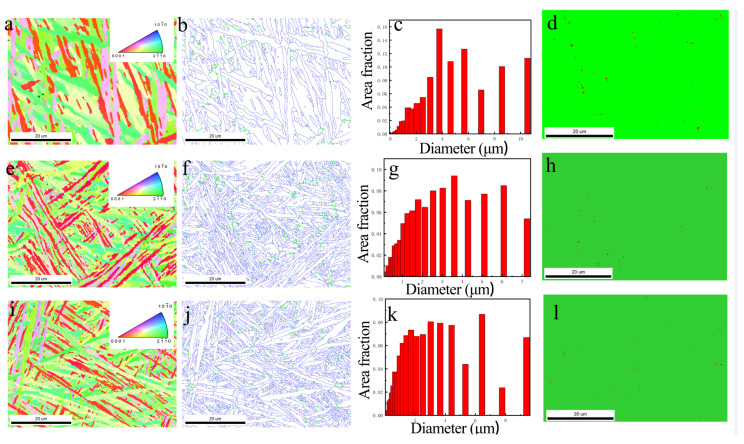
IPF maps, grain boundary maps, grain size distribution maps and phase proportion distribution maps of different ultrasonic frequency pulse currents: (a–d), conventional TIG; (e–h), ultrasonic frequency pulse current 6 A; (i–l), ultrasonic frequency pulse current 30 A.
